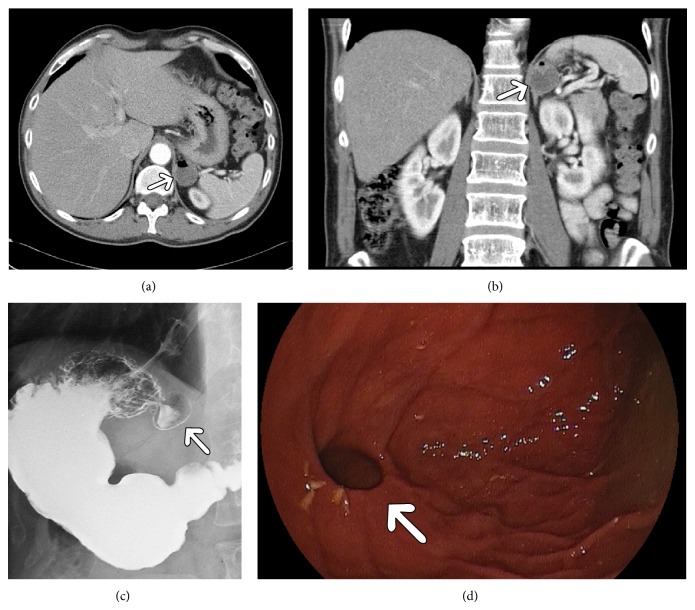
Imaging findings in a 57-year-old patient. (a) Computer tomographic scan detecting a mass in the left paravertebral region behind the stomach with air fluid level (arrow). (b) The lesion (arrow) in coronal CT reconstruction. (c) Upper gastrointestinal investigation with contrast showing a gastric diverticulum in the cardia on the posterior gastric wall below the esophagogastral junction (arrow). (d) Endoscopy finding with entry of the diverticulum (arrow).
